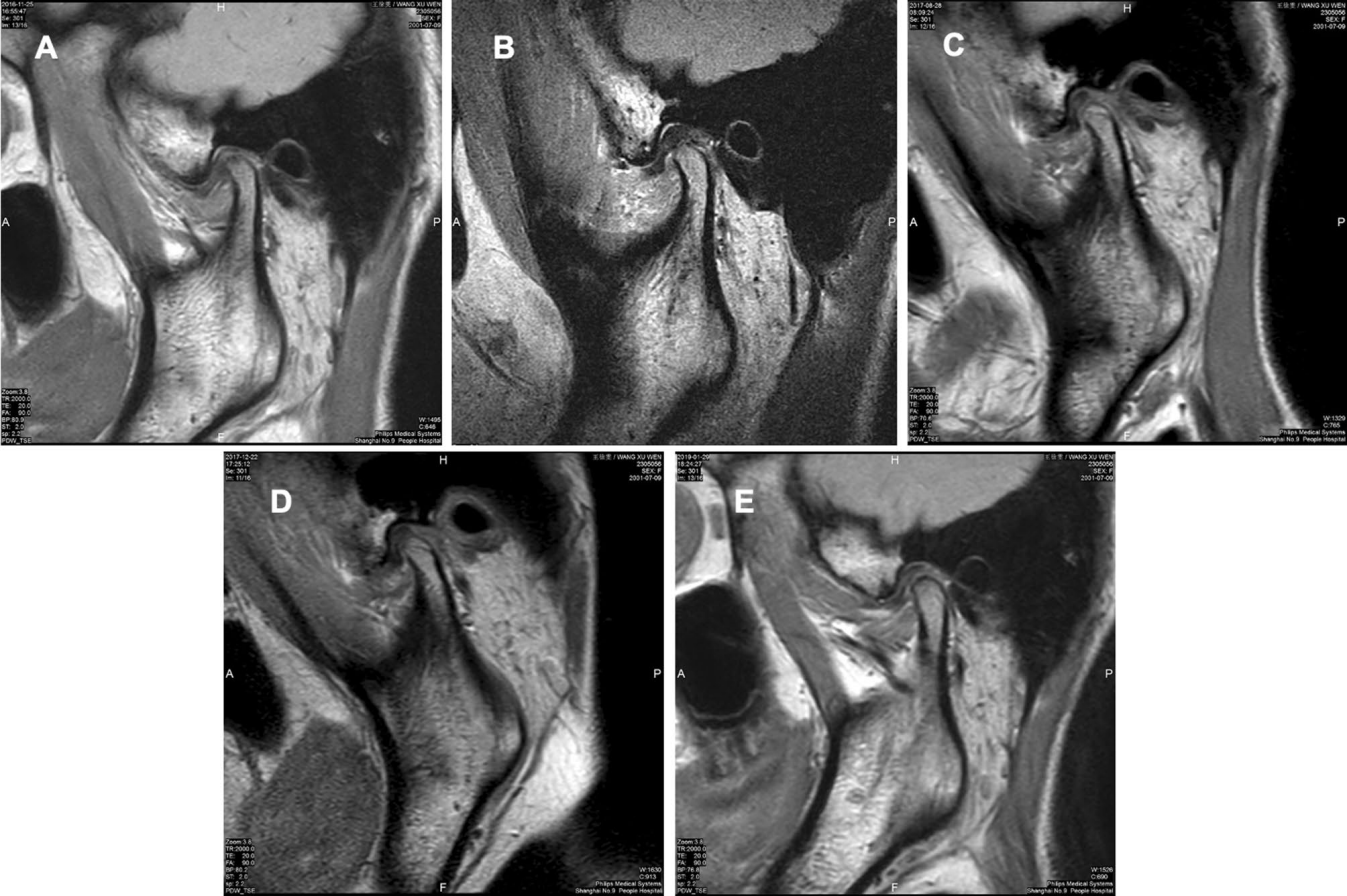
Typical case of new bone formation. (A) Pre-operative MRI showed TMJ ADDWoR. (B) Post-operative MRI showed well repositioned TMJ disc. (C) 6 months post-operatively, the disc was replaced and new bone was formed on the posterior slope of condyle. (D) 12 months post-operatively, newly formed bone reconstructed with the condyle. (E) 24 months post-operatively, the border between new bone and condyle vanished, the condyle was well remodeled.
